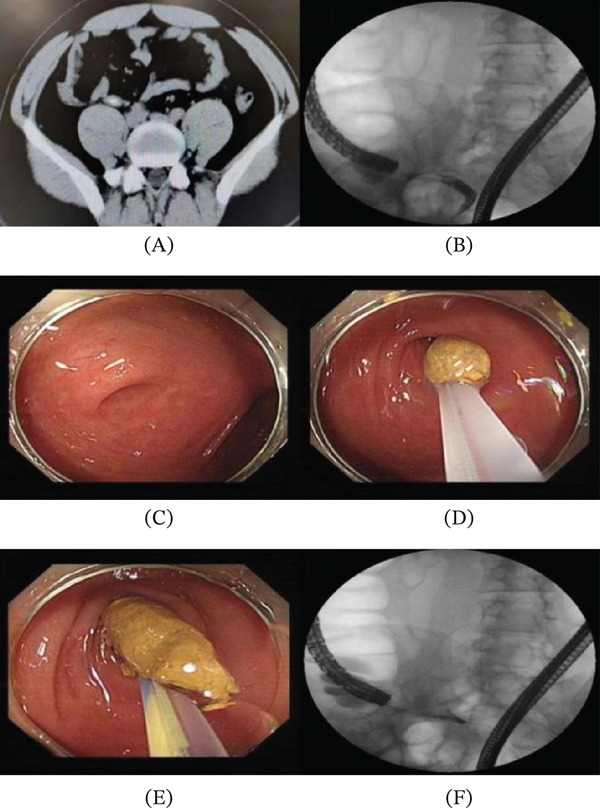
ERAT operation for chronic fecalith appendicitis. (A) Abdominal CT showed high‐density imaging in the appendiceal cavity; (B) X‐ray imaging revealed multiple filling defects in the appendix; (C) the colonoscopy indicated that the appendix opening was normal; (D + E) extraction of appendix fecalith; (F) X‐ray imaging showed no filling defect in the appendix.
